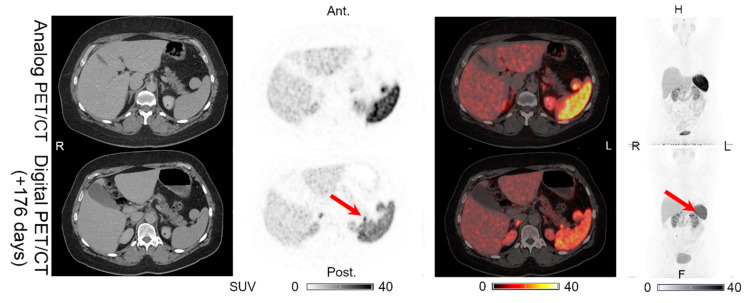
Patient 1: one additional pancreatic lesion found on the digital PET (without CT correlate) performed 176 days after the analog PET/CT, on which the lesion was not detectable. The CT of the digital PET/CT scan was performed without IV contrast. Arrow indicates lesion location.
